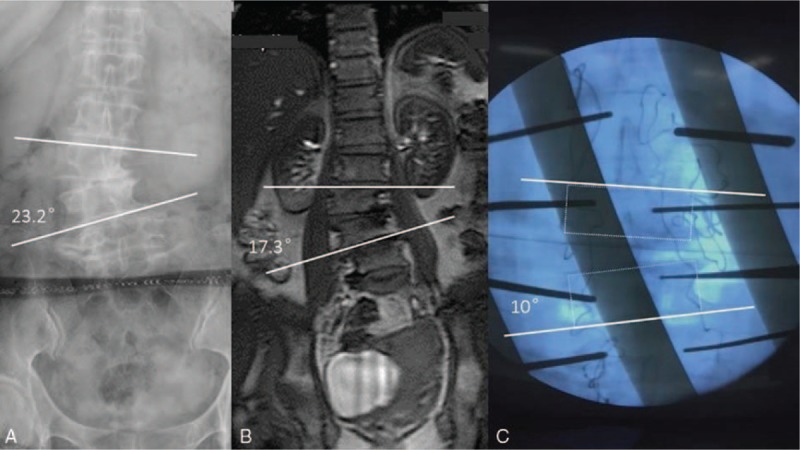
Radiographic example of a patient (female, 63 years) with degenerative lumbar scoliosis in 3 positions: (A) preoperative upright posteroanterior radiograph shows 23.2 degree of scoliosis; (B) in preoperative MR in supine position the Cobb angle reduced to 17.3 degree. (C) Positioned prone during the process of pedicle screw insertion from L2 to L5, the Cobb angle further reduced to 10 degree.
